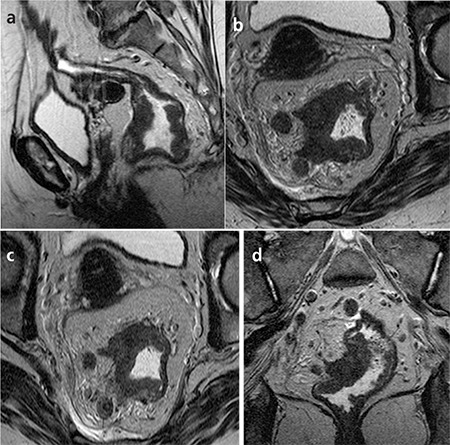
54-year-old woman with pT4N1 rectal cancer. Two observers staged the tumor as T3N2 in the preoperative MR imaging.
